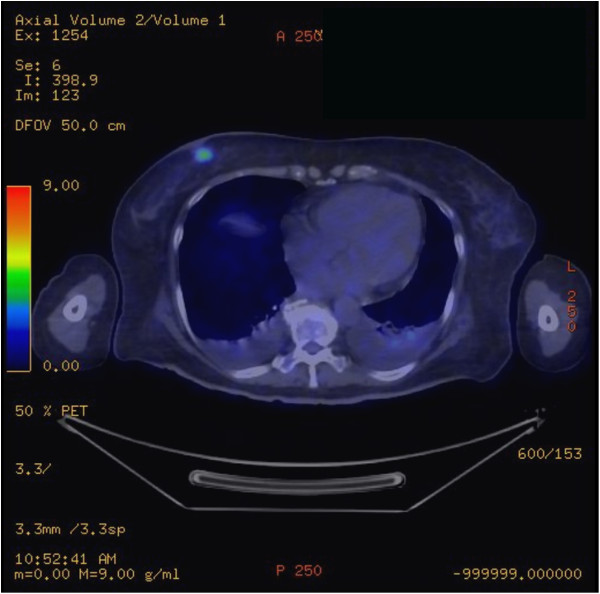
PET-CT. A 1.8 × 1.4 cm spiculated mildly FDG-avid right breast nodule just below the level of the nipple is demonstrated (maximum standardized uptake value 3.9). FDG, fluorodeoxyglucose; PET-CT, positron emission tomography-computed tomography.
